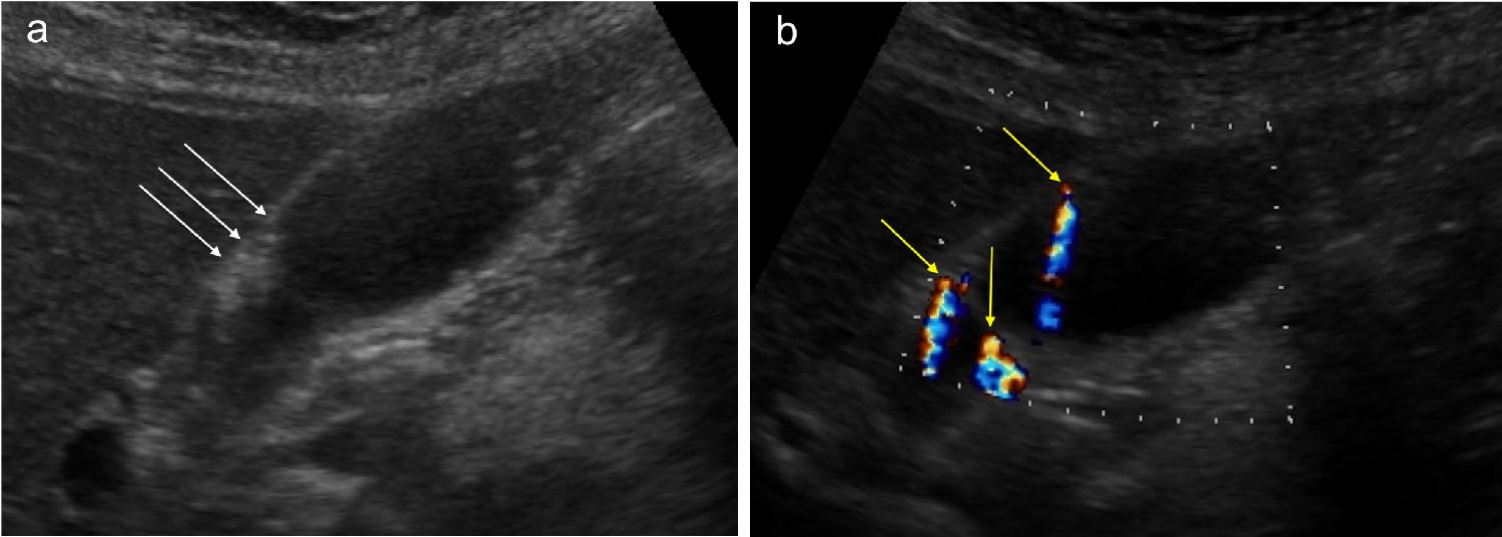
a Sonography image depicts parietal hyperechoic multiple foci on the gallbladder body and the infundibulum wall, generating comet-tail artifacts on B-mode (white arrows). b Sonography image depicts parietal hyperechoic multiple foci on the gallbladder body generating twinkling artifacts on the colour Doppler exam (yellow arrows)
